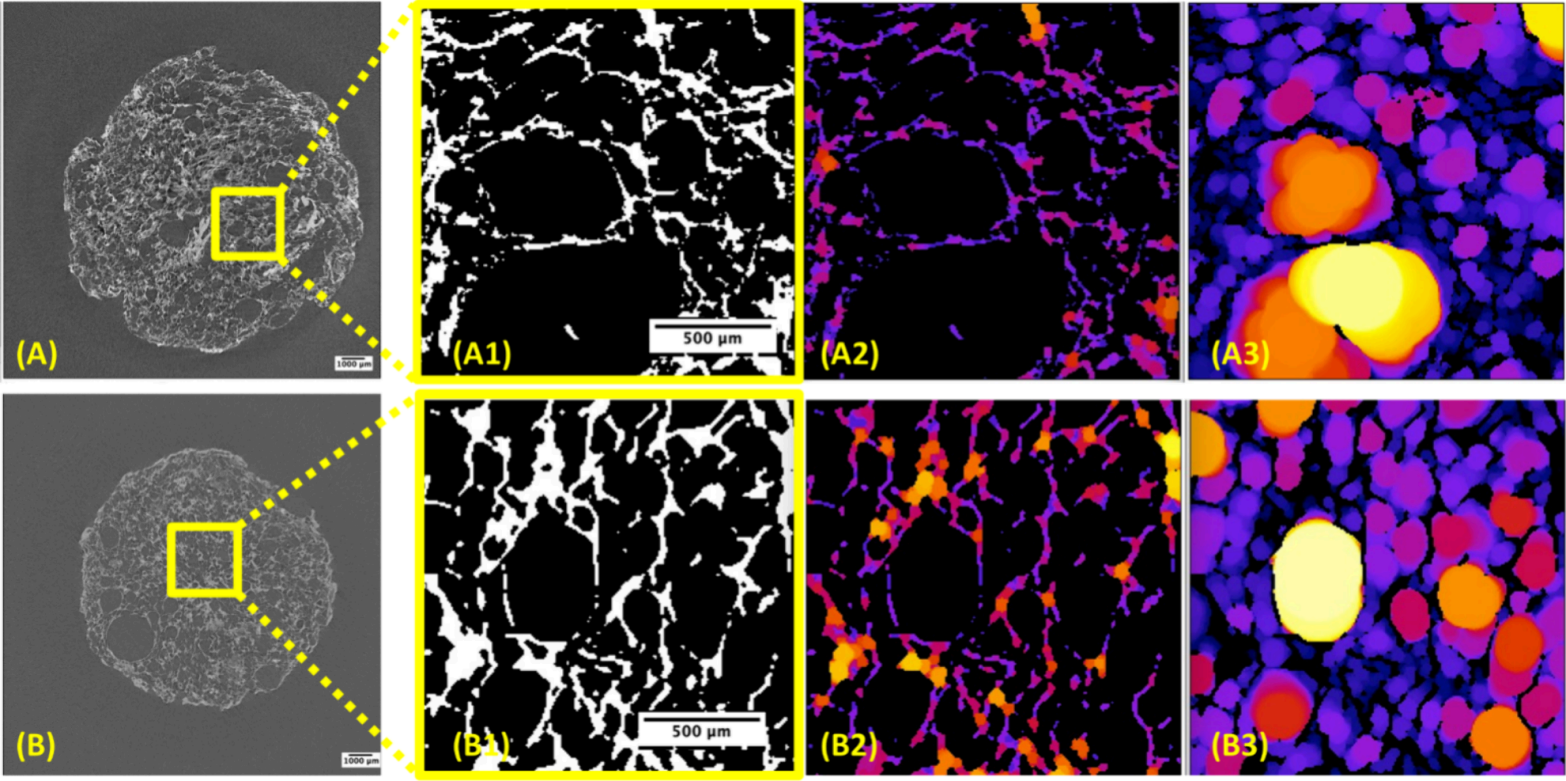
(A) μ-CT image of the SF/PEDOT:PSS/PVA 0.5% v/v foamed sample
